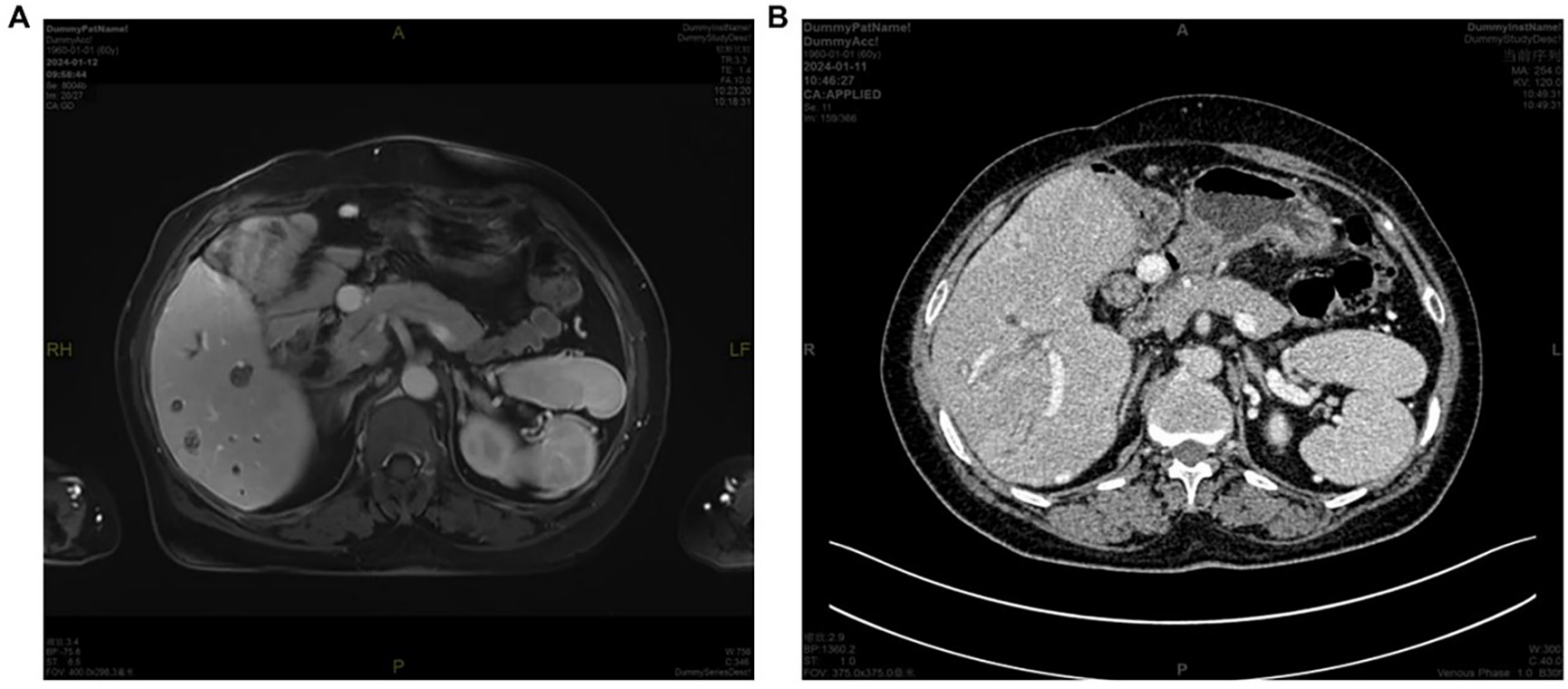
(A) MRI and (B) CT images demonstrating absence of the pancreatic tail.
